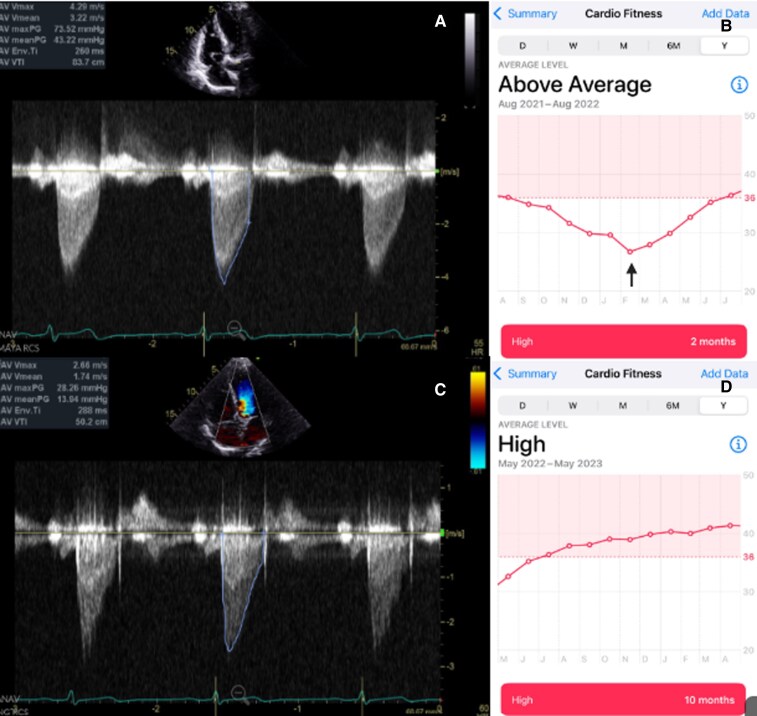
(*A*) Continuous-wave Doppler echocardiography shows high transvalvular velocity (4.29 m/s) and mean gradient of 43 mmHg, consistent with severe aortic stenosis. (*B*) Apple Watch cardiofitness application: estimated VO_2_ max trend showing progressive decline from approximately 36–26 mL/kg/min over months preceding TAVR. The nadir point (arrow) corresponds to the time of documented haemodynamic progression. X axis: time in months; Y axis: Apple Watch–estimated VO_2_ max in mL/kg/min. (*C*) Post-TAVR echocardiogram demonstrating markedly reduced transvalvular velocity (2.66 m/s) with a mean gradient of 13 mmHg, confirming relief of obstruction. (*D*) Subsequent Apple Watch–derived VO_2_ max trend demonstrating steady recovery and normalization of estimated VO_2_ max (∼41 mL/kg/min) during follow-up after valve replacement.
